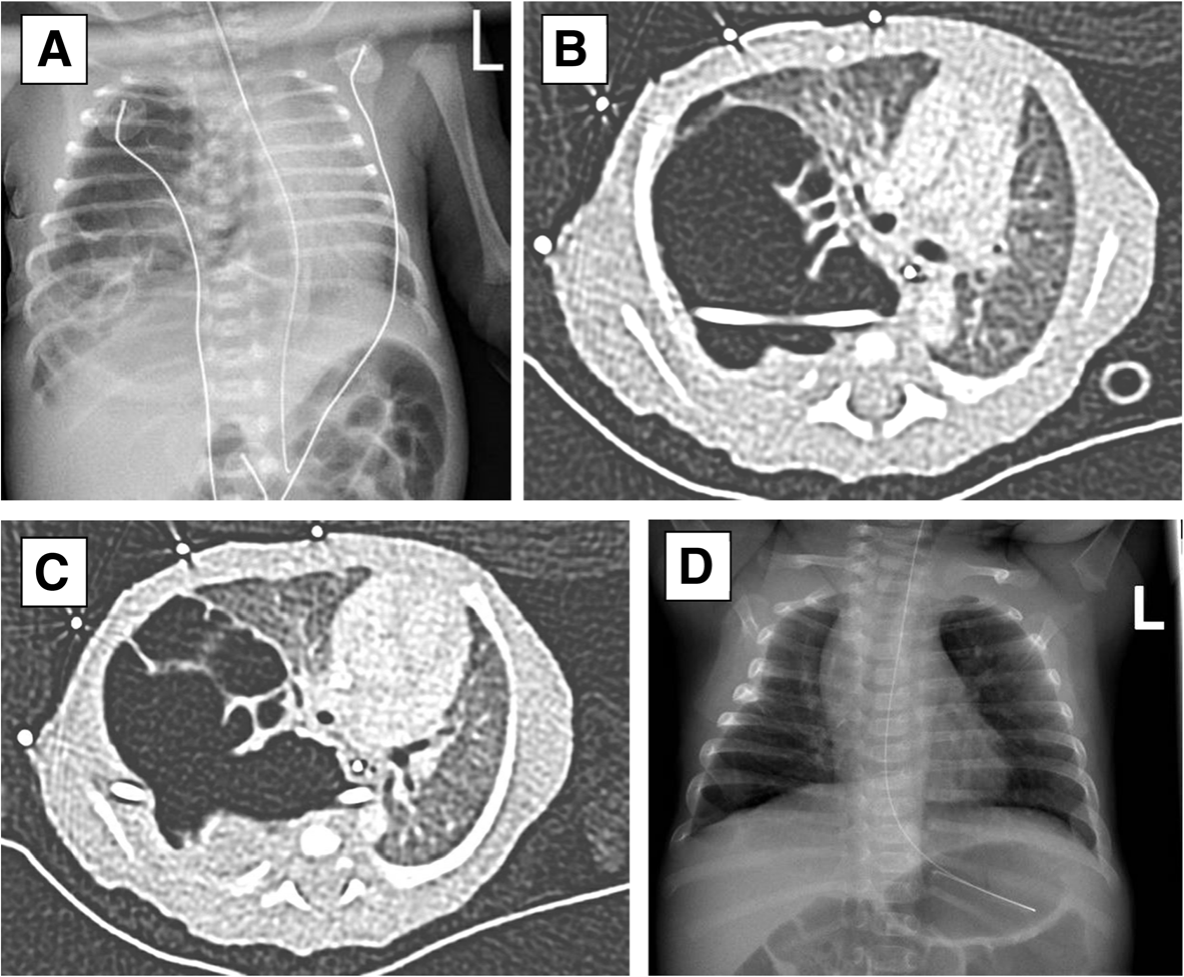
Chest X-ray after initial stabilization on the NICU ward a showed a large right lung with cyst-like appearance and a mediastinal shift to the left. High resolution chest CT scans b and c showed a thick walled multicystic air filled process with a left-sided mediastinal shift. The chest drain can be seen. Chest X-ray at the age of three months d showed slight post-operative thoracic cage deformity on the right, a clip in the right hilum, and normal aeration of both the right and the left lung
